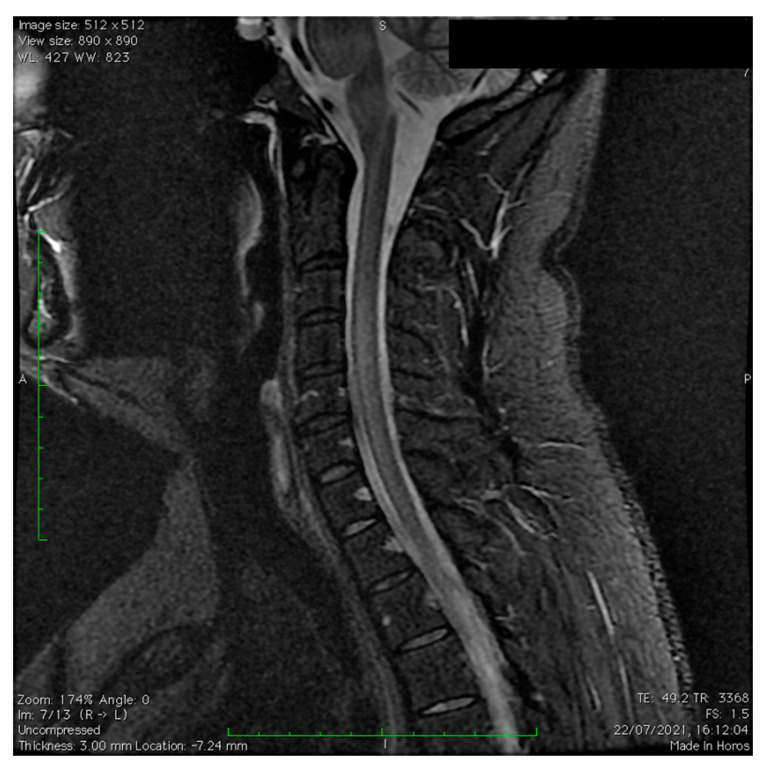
Sagittal spinal cord MRI, STIR sequences. Longitudinally extensive lesion at the level of the cervical spinal cord.
